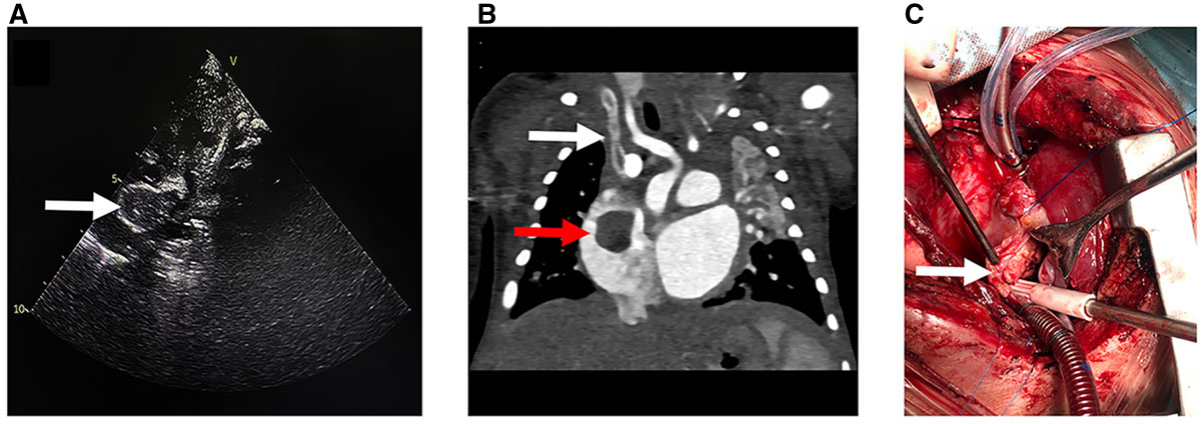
Imaging and surgery pictures. (A) Transthoracic echocardiogram demonstrating a large right atrial mass (arrow). (B) Right atrial mass (red arrow) and central venous catheters (white arrow) as seen on computed tomography (CT). (C) A right atrial thrombus was seen during surgery (arrow).
